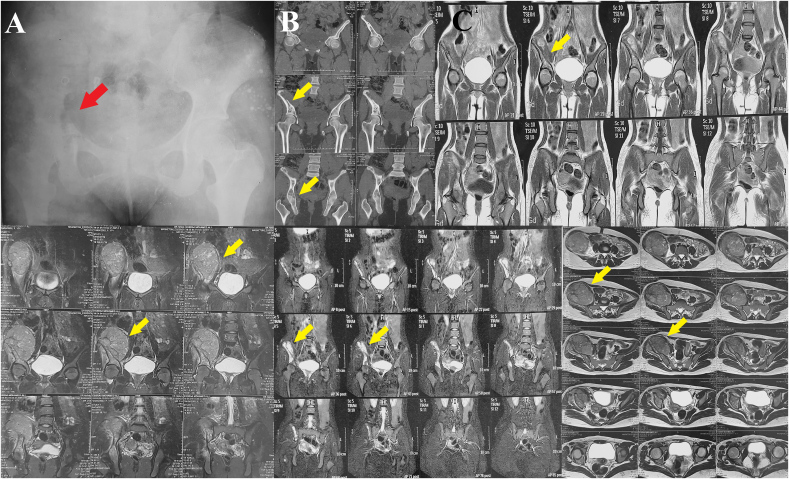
Preoperative imaging studies. A: pelvis anteroposterior plain radiographic view showing erosion of the inner aspect of the right acetabulum (red arrow). B: a CT scan of the pelvis showing affection of the right iliac bone (yellow arrow). C: Different pelvis MRI cuts showing a soft tissue mass arising within the iliacus muscle in the medial to the right iliac bone (yellow arrows). (For interpretation of the references to colour in this figure legend, the reader is referred to the web version of this article.)
